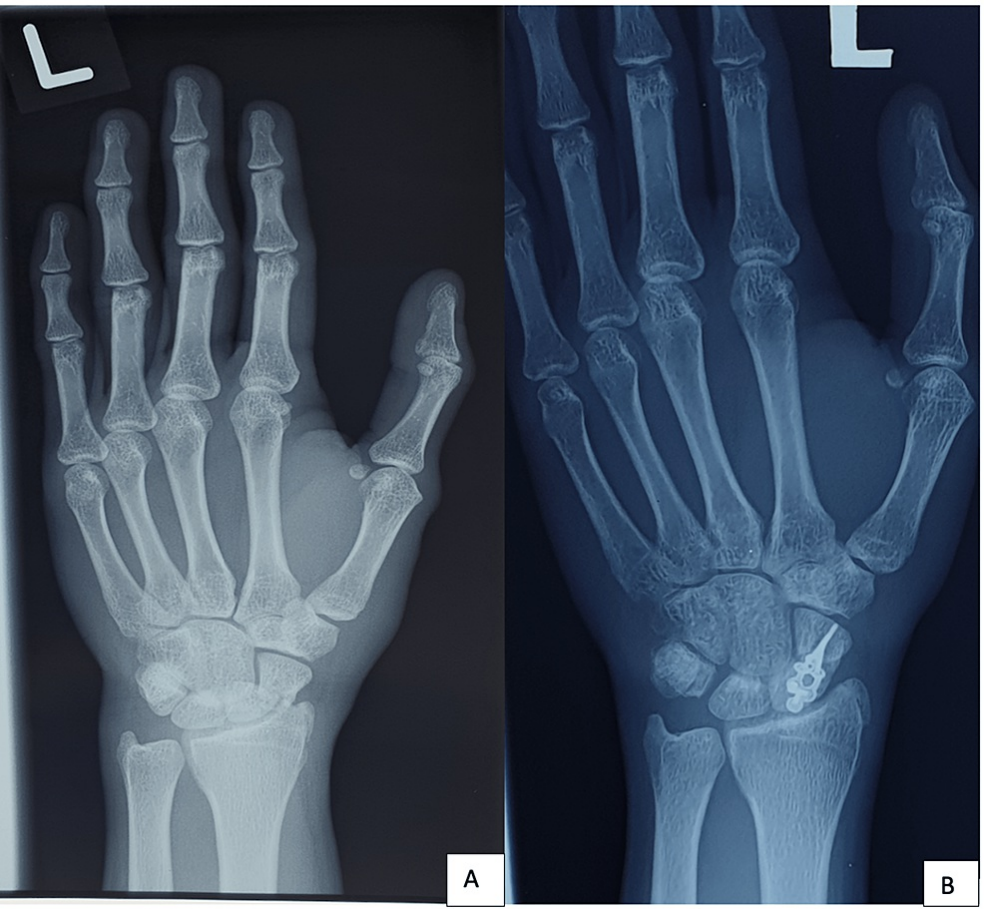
A. Preoperative radiograph: transradial transscaphoid perilunate dislocation; B. 1-year postoperative radiograph
